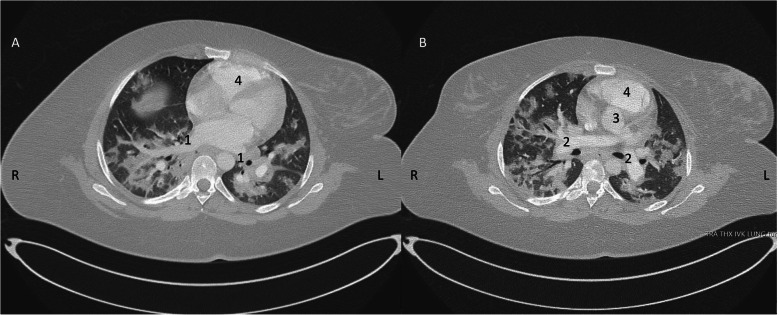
Computed tomography (CT) thorax scan showing bilateral diffuse, ground-glass opacities with both peripheral and perihilar distribution and no signs of pulmonary embolism. Two segments from the same session post-partum on day 2 of readmission (22/4/2020). 1 Pulmonary vein. 2 Pulmonary artery. 3 Aorta. 4 Right ventricle
